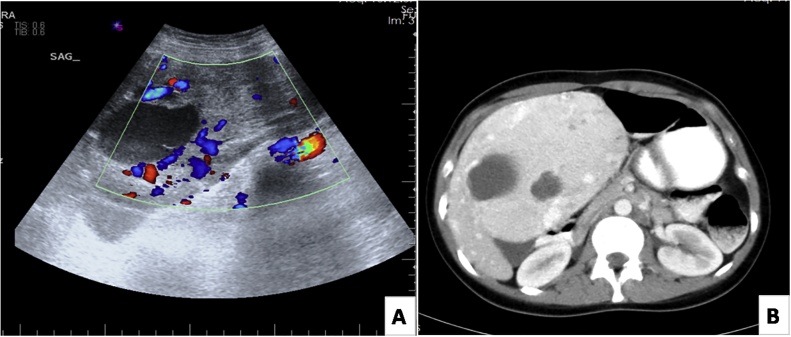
(A) Doppler ultrasonogram showing hyperechogenic, cystic, and hypervascular hepatic perivascular epithelioid cell tumor. (B) Computed tomography scan of tumor.
